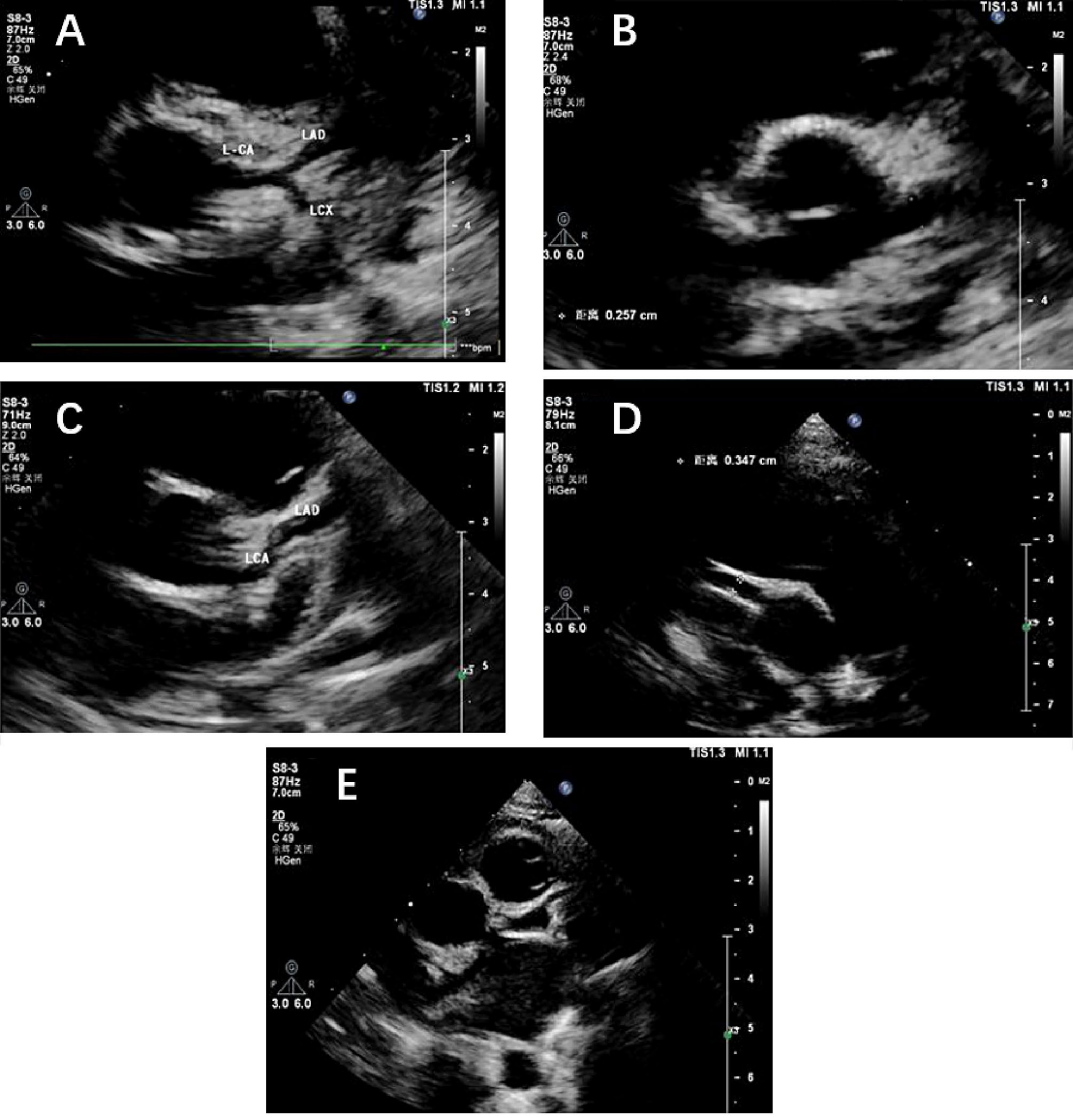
The figures of echocardiography. (A) Normal; (B) Dilatation of the left main coronary artery; (C) Dilatation of the left main coronary artery and the left anterior descending branch with thrombus formation; (D) Dilatation of the right coronary artery; (E) Localized aneurysm-like dilatation of the left anterior descending coronary artery
